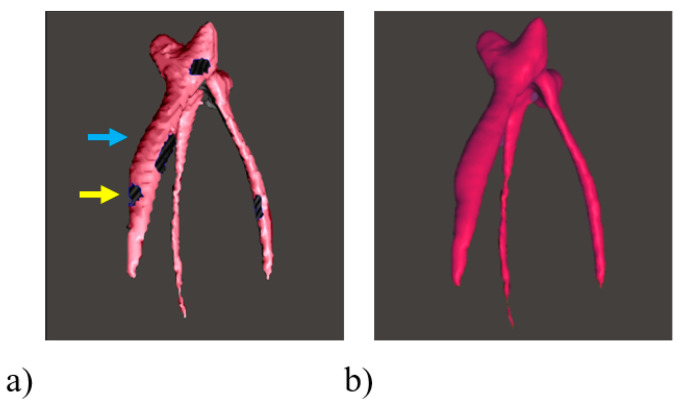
Cleaning and repairing the 3D file of a segmented pulp using the free software Meshmixer (Autodesk, San Rafael, EU). (a) STL presenting holes (yellow arrow) and stair-steps (blue arrow) due to the resolution of the cone beam computed tomography, could be automatically smoothed and repaired using “Smooth” and “Inspector” tools in the software (b).
